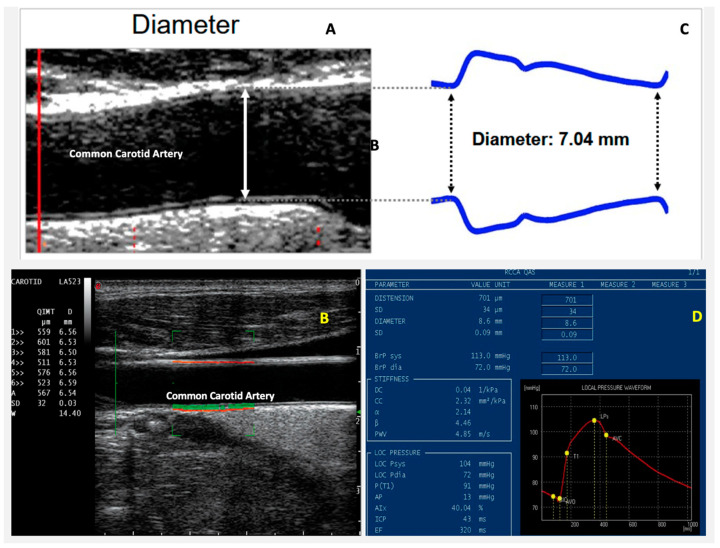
Vascular morphologic and functional analysis. Radio Frequency-Quality Intima Media Thickness (^RF^QIMT): during the scanning of the carotid artery a real-time feedback on measurement quality via quality indicators overlaid on the ultrasound image at the position of the vessel wall (orange lines) and the far wall intima layer (green line) (A,B). Radio Frequency Quality Arterial Stiffness (^RF^QAS) targets the measurement of the blood vessel stiffness of a subject in a selected area of investigation. The blood vessel wall stiffness is expressed as pulse wave velocity obtained from brachial blood pressure and the accurate measurements of diameter and change in diameter. Moreover, the local blood pressure at the site of the ultrasound measurement is given (C,D). ^RF^QIMT and ^RF^QAS, respectively—Esaote.
